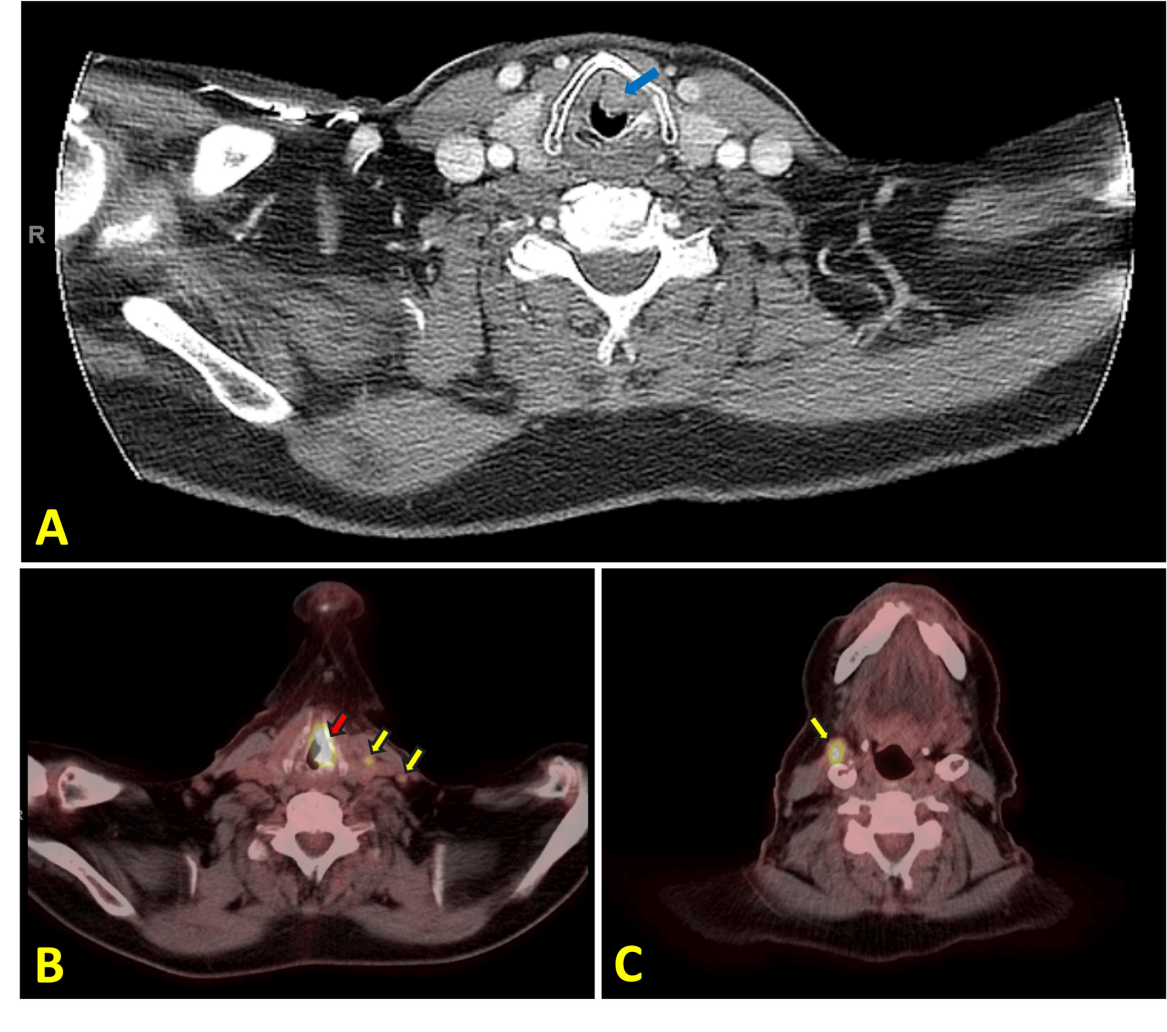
A: CT scan of the neck with a blue arrow indicating the polypoid lesion of the left vocal cord. B: PET-CT scan of laryngeal cancer with a red arrow showing FDG uptake at the left vocal cord and yellow arrows showing two normal-sized FDG-avid lymph nodes. C: PET-CT scan of neck with a yellow arrow showing a right upper anterior cervical FDG-avid lymph node, measuring 1.3 cm PET-CT: Positron Emission Tomography-Computed Tomography; FDG: Fludeoxyglucose
